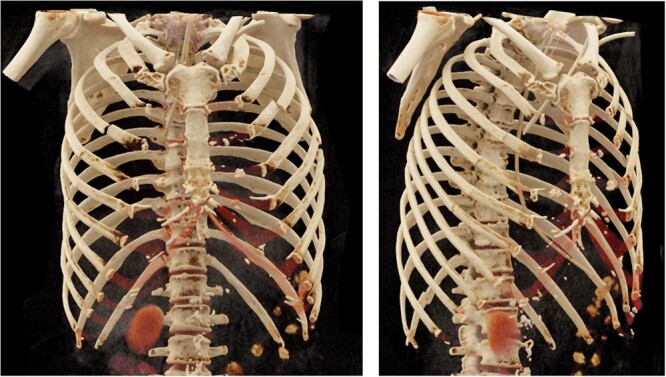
Three-dimensional reconstruction of post-cardiopulmonary resuscitation computed tomography scan chest: rib fractures 2–7 (5–7 multifragmented) and 12 right, 2–7 left, transverse sternal body fracture, clavicle shaft fracture (between mid and lateral third of clavicle, not visible in reconstruction).
